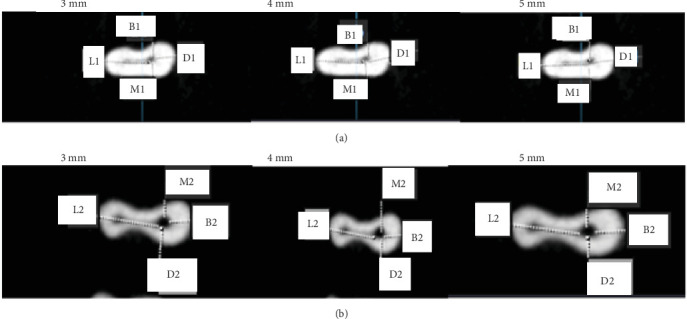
Cone beam computed tomography (CBCT) images before (A) and after (B) instrumentation of the mesiobuccal canal by ProTaper Next (PTN) at 3, 4, and 5 mm from the apex.
